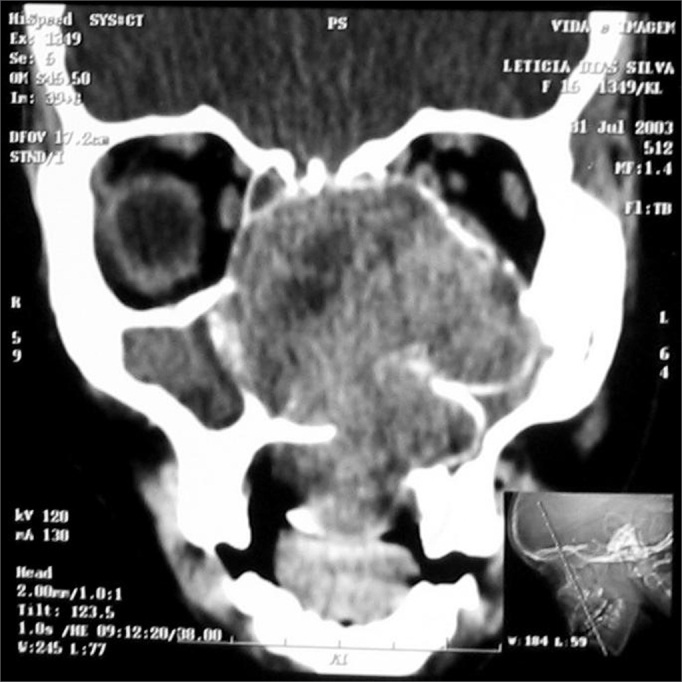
CT scan - coronal view of a nasosinusal hemangiopericytoma (case 4).
